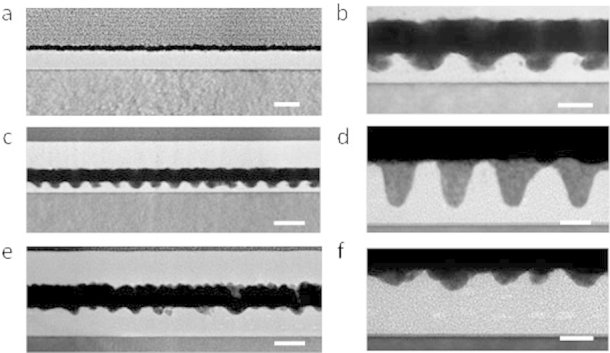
Cross-sectional TEM images of (a) solvent annealed film for 16 k–5 k and nanoporous template after ethanol ultrasonication for different time (b), (c) 32 k–11 k, 15 min (d) 16 k–5 k, 10 min (e), (f) 16 k–5 k, 5 min respectively. (a), (c), (e) scale bar: 50 nm. (b), (d), (f) scale bar: 20 nm.
